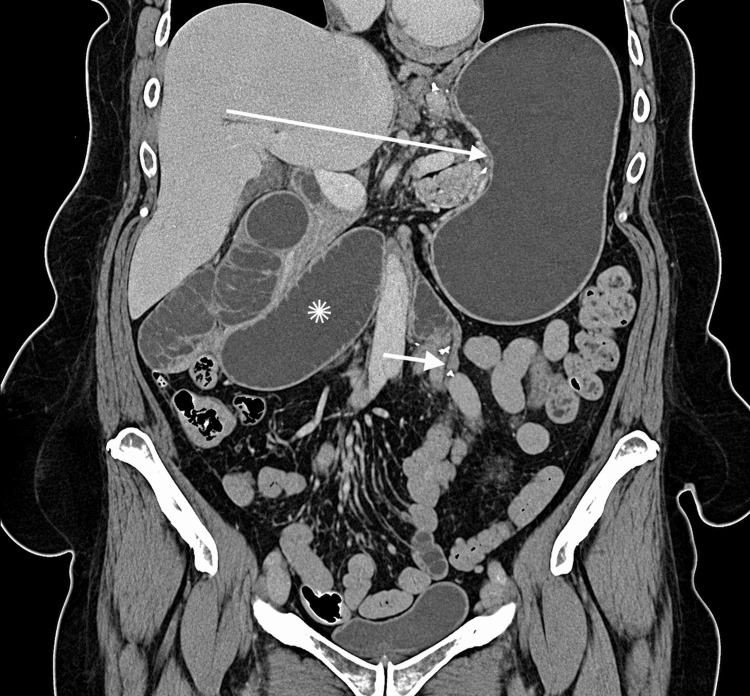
Pre-procedural coronal CT demonstrating gastric remnant distension Coronal CT image demonstrating marked distension of the excluded stomach (white arrow) and dilatation of the biliopancreatic limb (asterisk) secondary to stenosis of the proximal jejunojejunal anastomosis (short white arrow) in a patient with prior Roux-en-Y gastric bypass.
